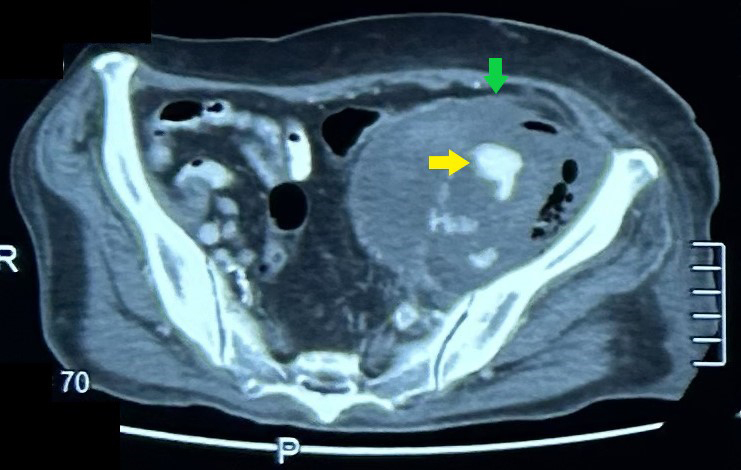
CT abdomen revealed a multiloculated abscess involving the left iliopsoas muscle (green arrow), with a pseudoaneurysm arising from the left external iliac artery (yellow arrow). CT: computed tomography.
